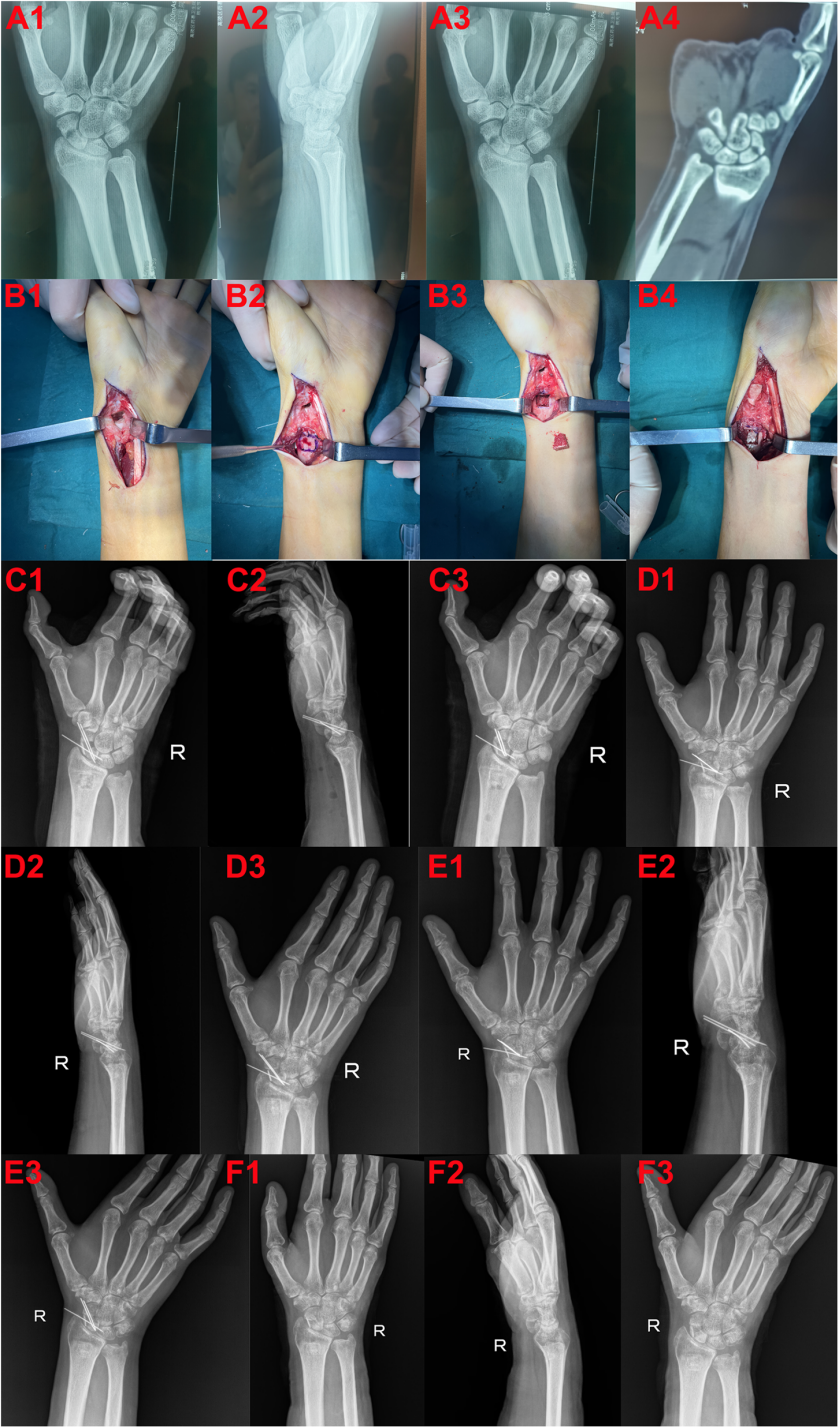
A 27-year-old male patient who underwent surgery. (A1–3) Preoperative radiographs of the wrist in anteroposterior, lateral, and ulnar deviation views. (A4) Preoperative CT scan showing scaphoid nonunion. (B1) Intraoperative image depicting debridement of the scaphoid fracture site. (B2) Location of the distal radius where autologous bone graft was harvested. (B3) Harvested autologous bone from the distal radius. (B4) Implantation of autologous bone graft into the scaphoid nonunion site. (C1–3) Postoperative radiographs of the wrist in anteroposterior, lateral, and ulnar deviation views. (D1–3) Radiographs of the wrist one month postoperatively in anteroposterior, lateral, and ulnar deviation views. (E1–3) Radiographs of the wrist two months postoperatively in anteroposterior, lateral, and ulnar deviation views. (F1–3) Radiographs following removal of internal fixation, showing anteroposterior, lateral, and ulnar deviation views.
